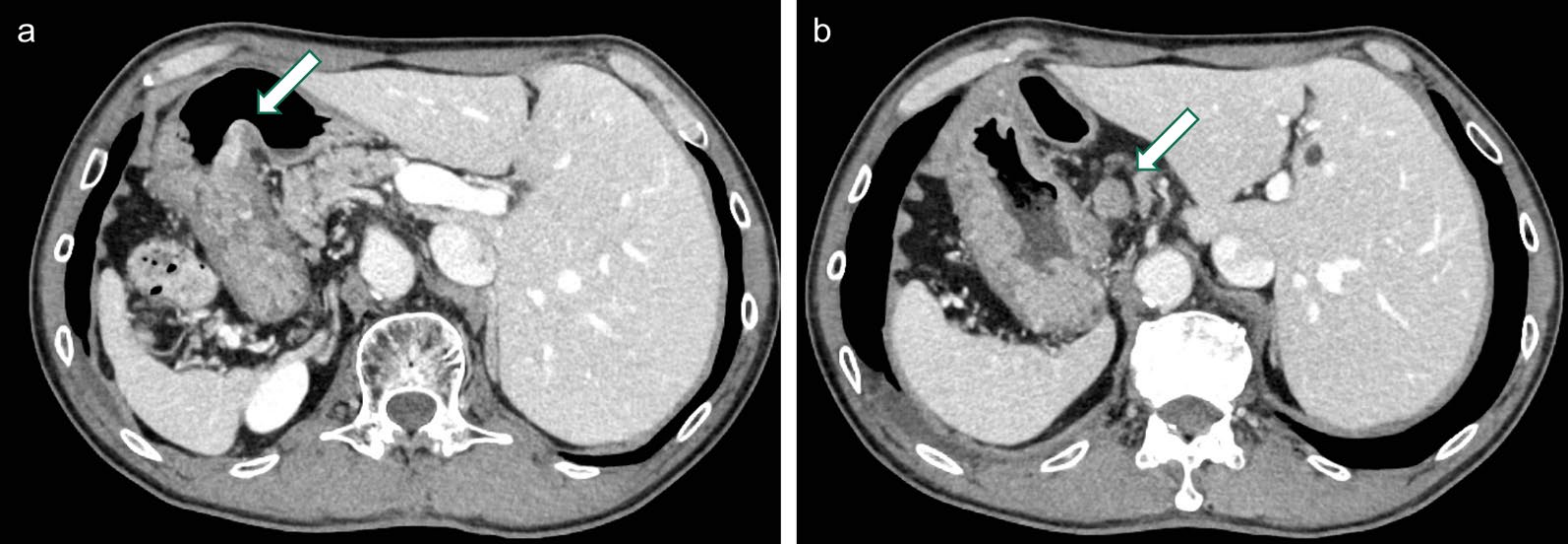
Computed tomography (CT) findings. CT showed complete transposition of the abdominal organ, irregular thickening of the gastric wall (a, arrow), and metastasis to lymph node #7 (b, arrow)
